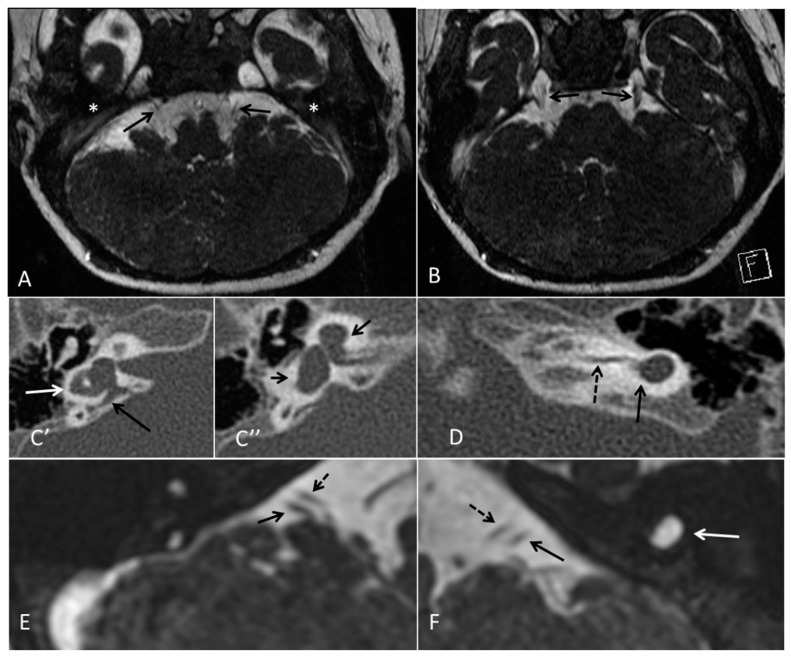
Axial T2 high resolution 3D sequence on the internal auditory canal (IAC) in patient 1 (A,B) show absence of membranous labyrinth in both petrous bones (*) with presence of VII nerves (arrows in A) and V nerves (arrows in B). Axial CT petrous bone in patient 7 shows incomplete partition type 1 malformation on the right (C′,C″), characterised by dysplastic lateral semicircular canal (white arrow in C′), dilated vestibular aqueduct (black arrow in C′), dilated cochlea and vestibule without visualisation of the modiolus (arrows in C″). In the same patient on the left (D) there is a rudimentary otocyst (arrow) with very narrowed IAC (dotted arrow). Axial T2 high resolution 3D sequence on the IAC in patient 7 (E,F) demonstrates presence of VII (dotted arrow) and VIII (black arrow) cranial nerves in the ponto-cerebellar angle on both sides. The rudimentary otocyst on the left is also noted (F, white arrow).
